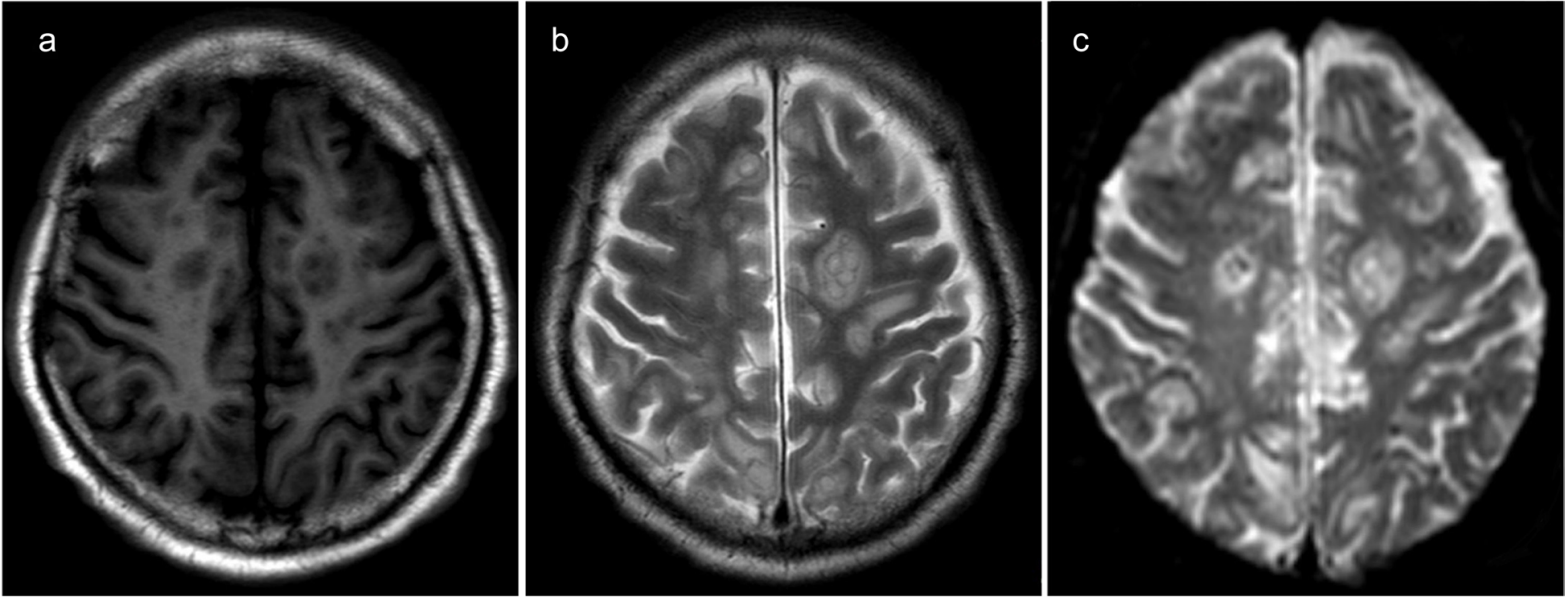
On day 21, the brain magnetic resonance imaging (MRI) revealed widespread plaques or circular abnormal signals within the brain parenchyma. These signals appeared as low intensity on T1-weighted imaging (a), high intensity on T2-weighted imaging (b), and showed restricted diffusion on diffusion-weighted imaging (c)
